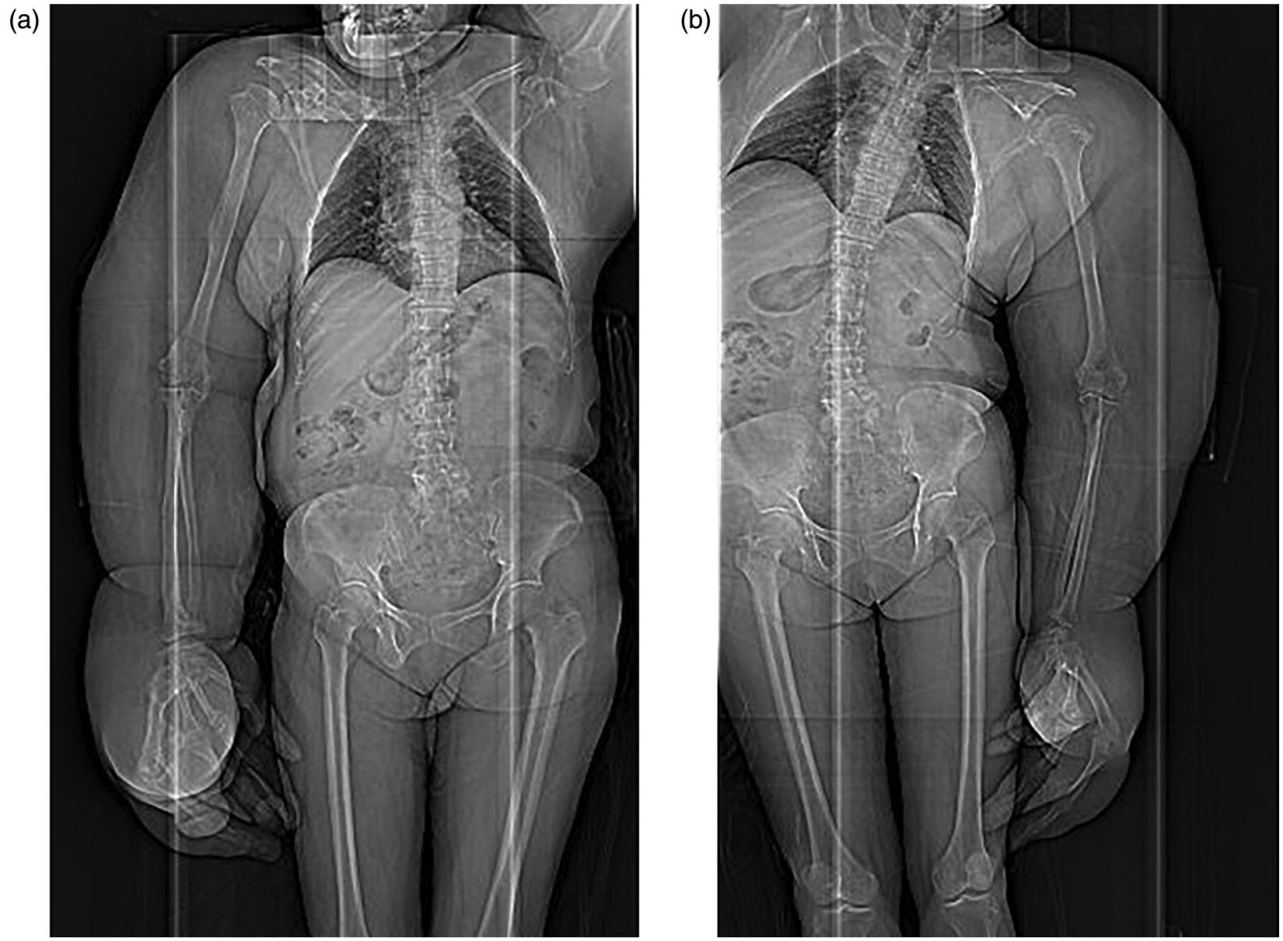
(a,b) CT scans: Any congenital anomalies (e.g., syndactyly) were not found, except the bilateral, disproportional overgrowth of the upper extremities.
